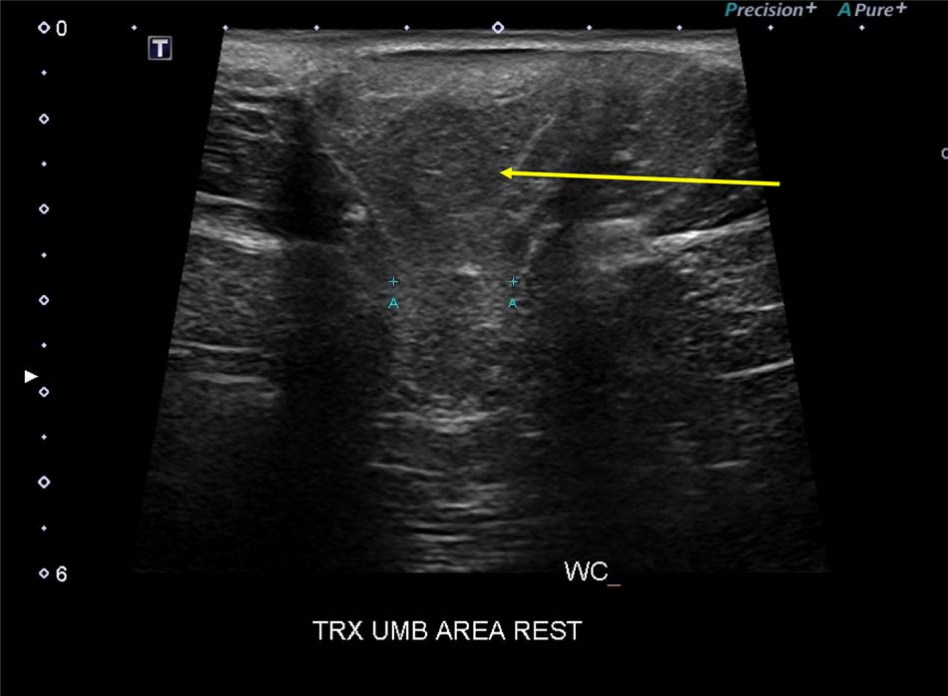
Abdominal wall ultrasound showing the one parietal peritoneal lipoma in the hernia sac (yellow arrow) and the 2 cm hernia neck (A-A).
